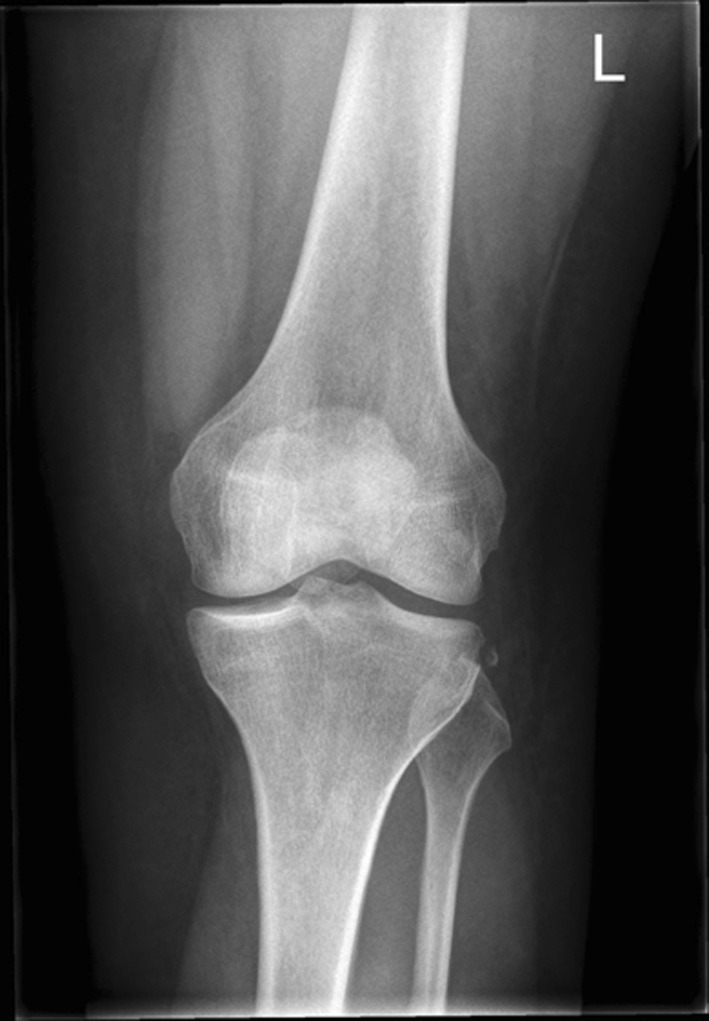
Anteroposterior X-ray indicating a Segond fracture
